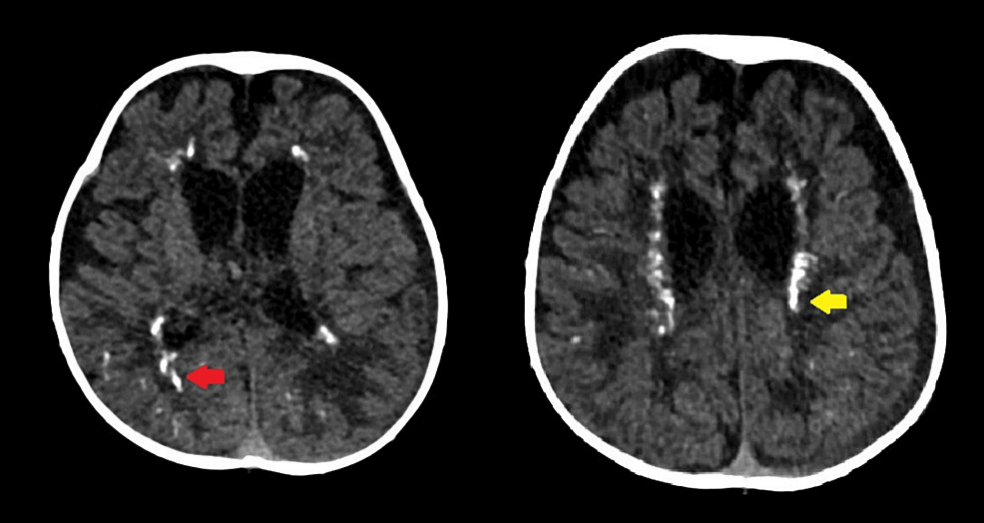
Axial CT images of the brain showed linear hyperdensities in the subcortical white matter of bilateral parietal lobes (red arrow) and periventricular regions (yellow arrow) suggestive of intracranial calcifications. Ventricles appeared mildly dilated. Increased subarachnoid space was noted in bilateral frontoparietal regions suggestive of benign enlargement of the subarachnoid space in infancy.
